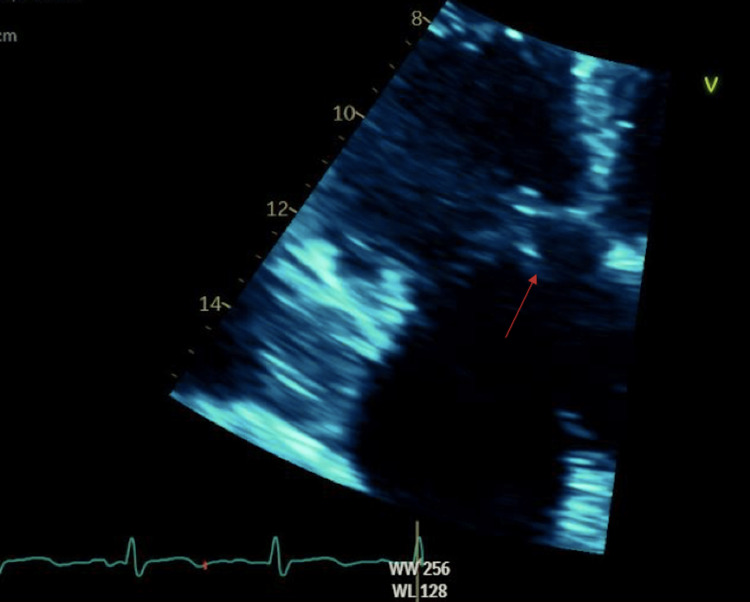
TTE image of the tricuspid vegetation. TTE, transthoracic echocardiogram
